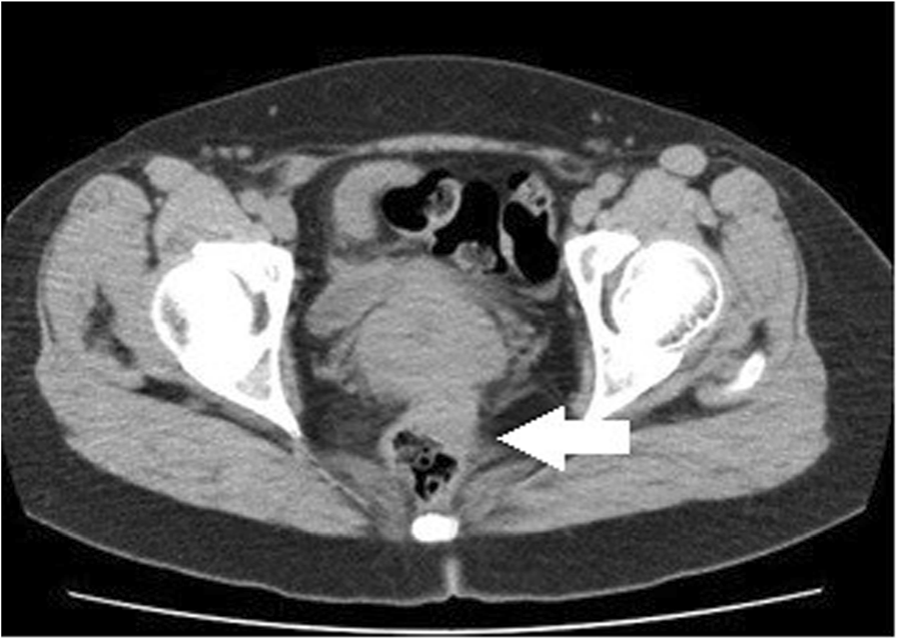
Computed tomography shows the 40-mm mass on the anterior wall of the rectum
